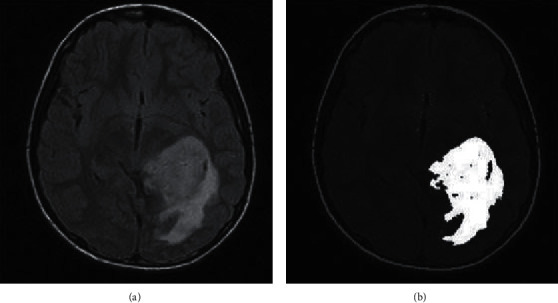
MRI image instance (a) and its segmented region using GA (b).
